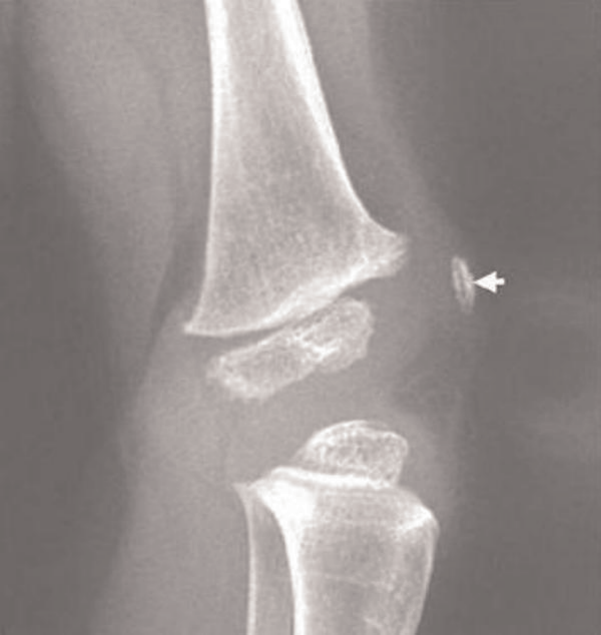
Lateral radiograph of the knee showed unusual early ossification of the patella.
